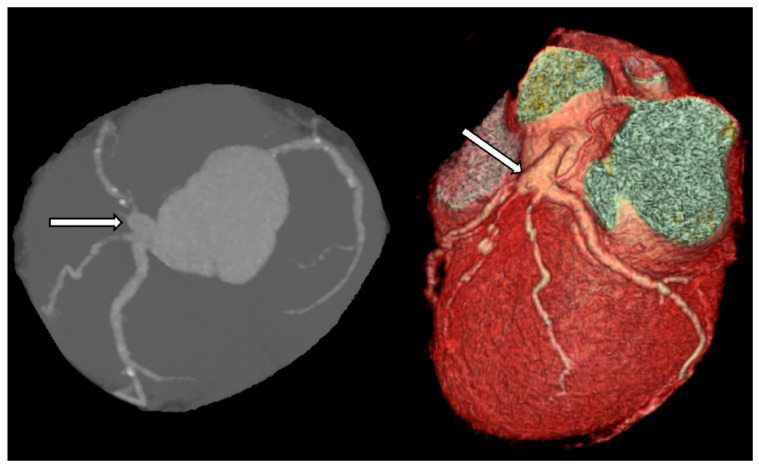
The aneurysmal lesion (arrow) of the left main coronary artery in CT.
